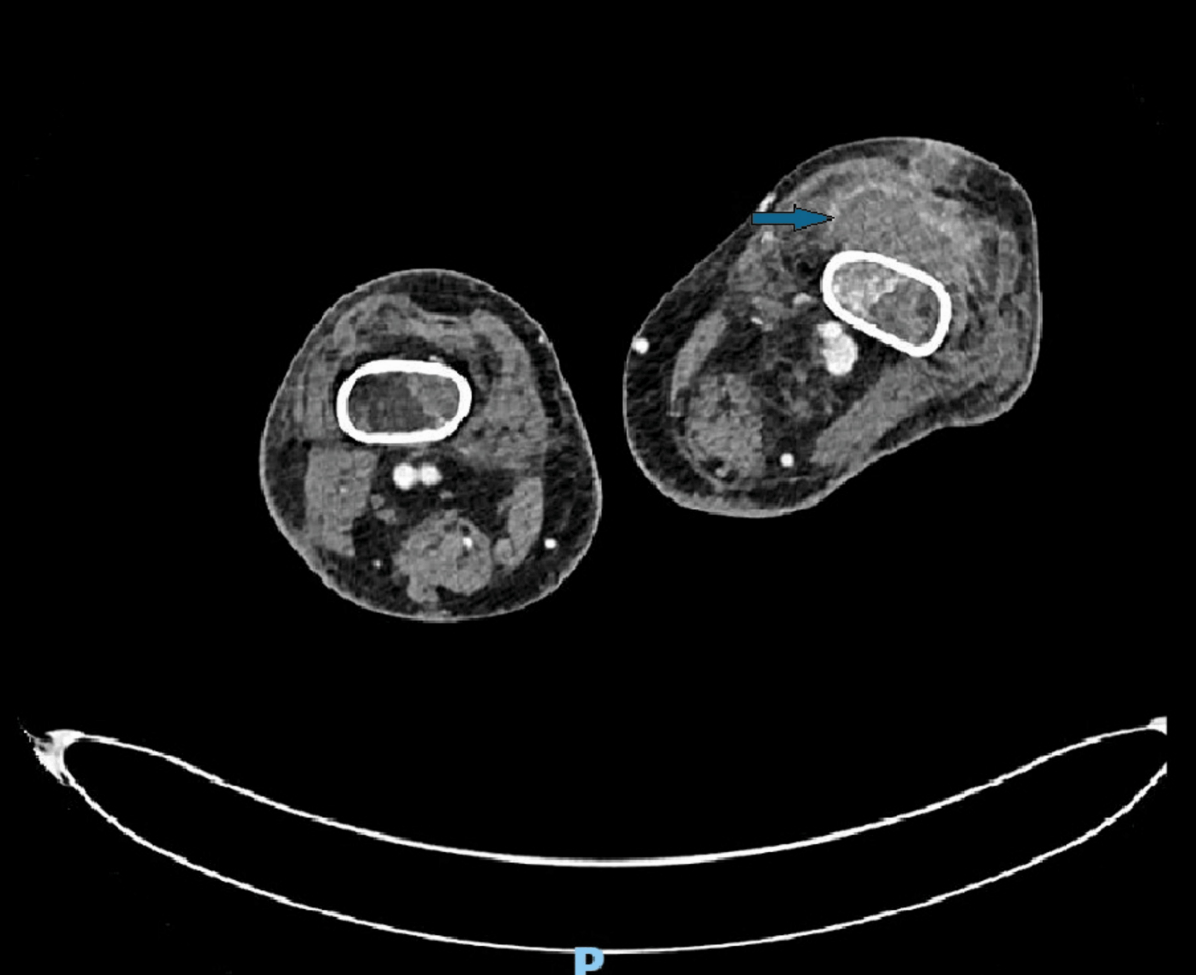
CT scan showing one of multiple muscular abscesses.
